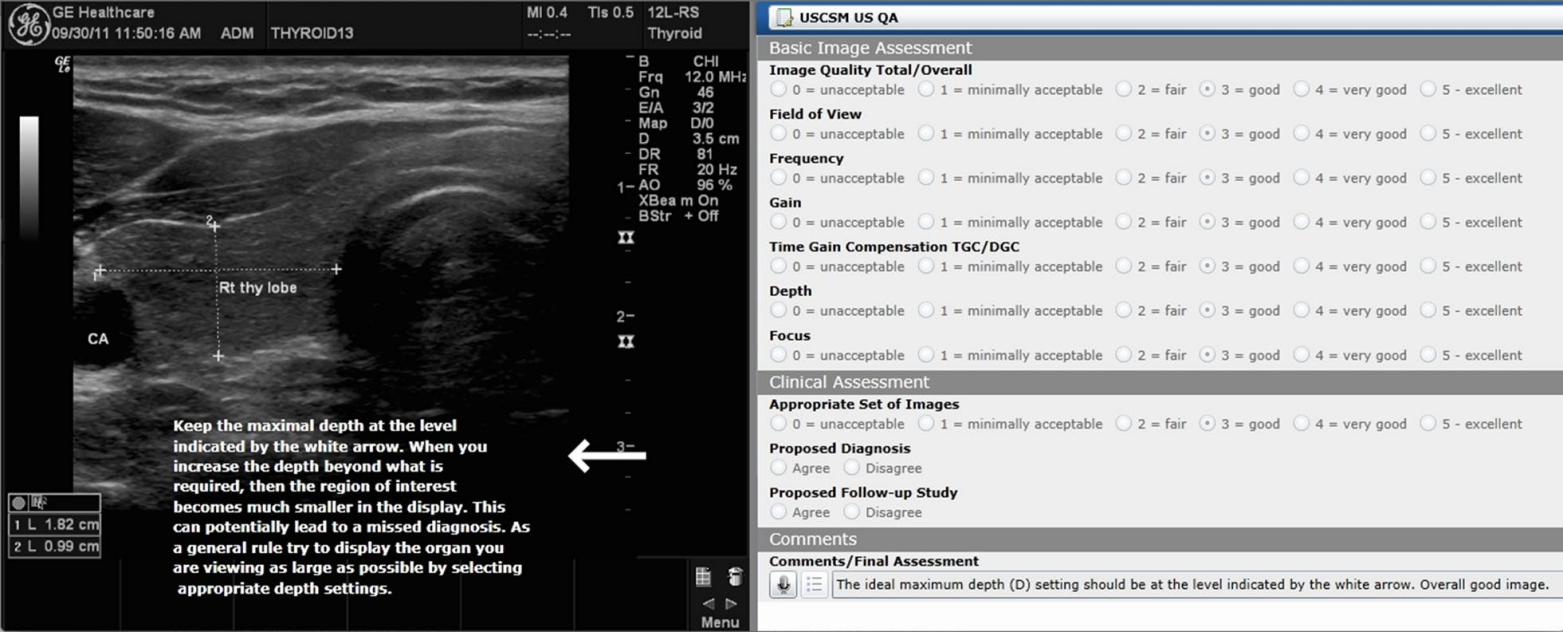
Student ultrasound image evaluation via cloud-based review portal
